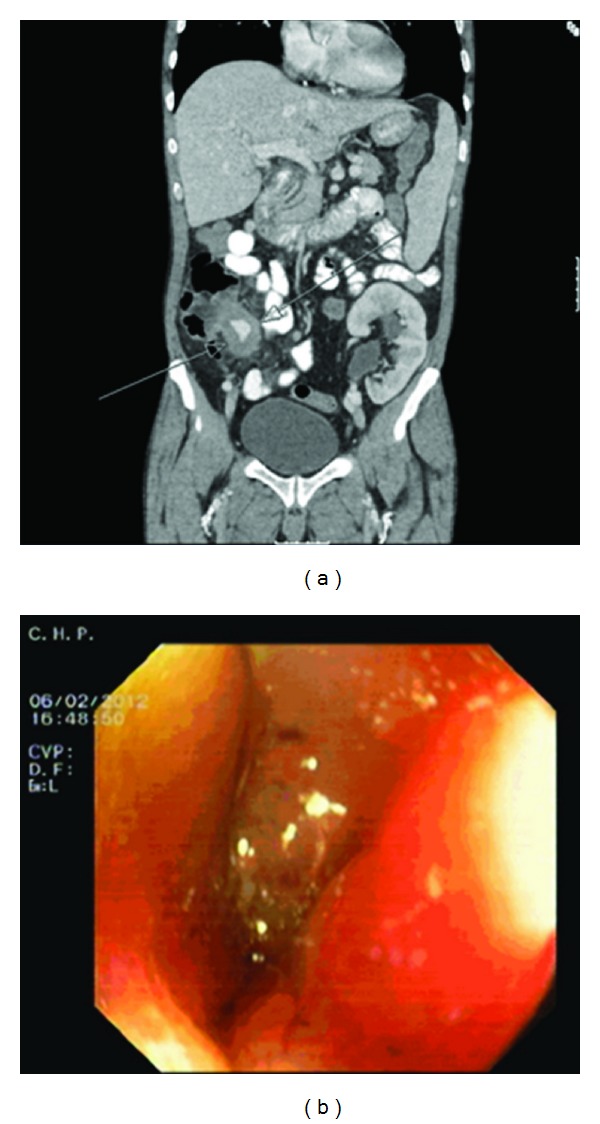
(a) Abdominal CT showing terminal ileitis. (b) Colonoscopy showing congestion and hyperemia of ileocecal valve.
